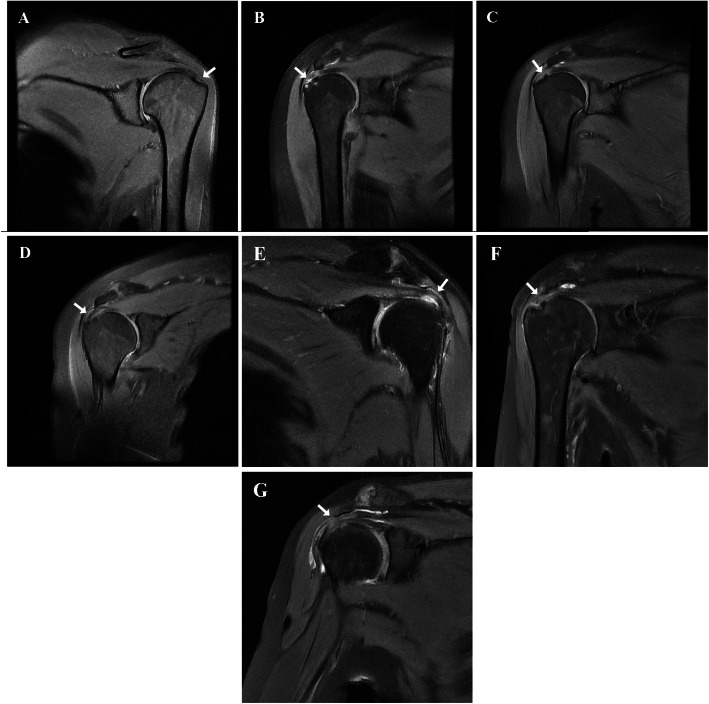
a–g Cases 1 ~ 4, 6, and 8 ~ 9: Preoperative sagittal T2-weighted magnetic resonance (MR) imaging reveals the interruption of supraspinatus tendon continuity (white arrows)
